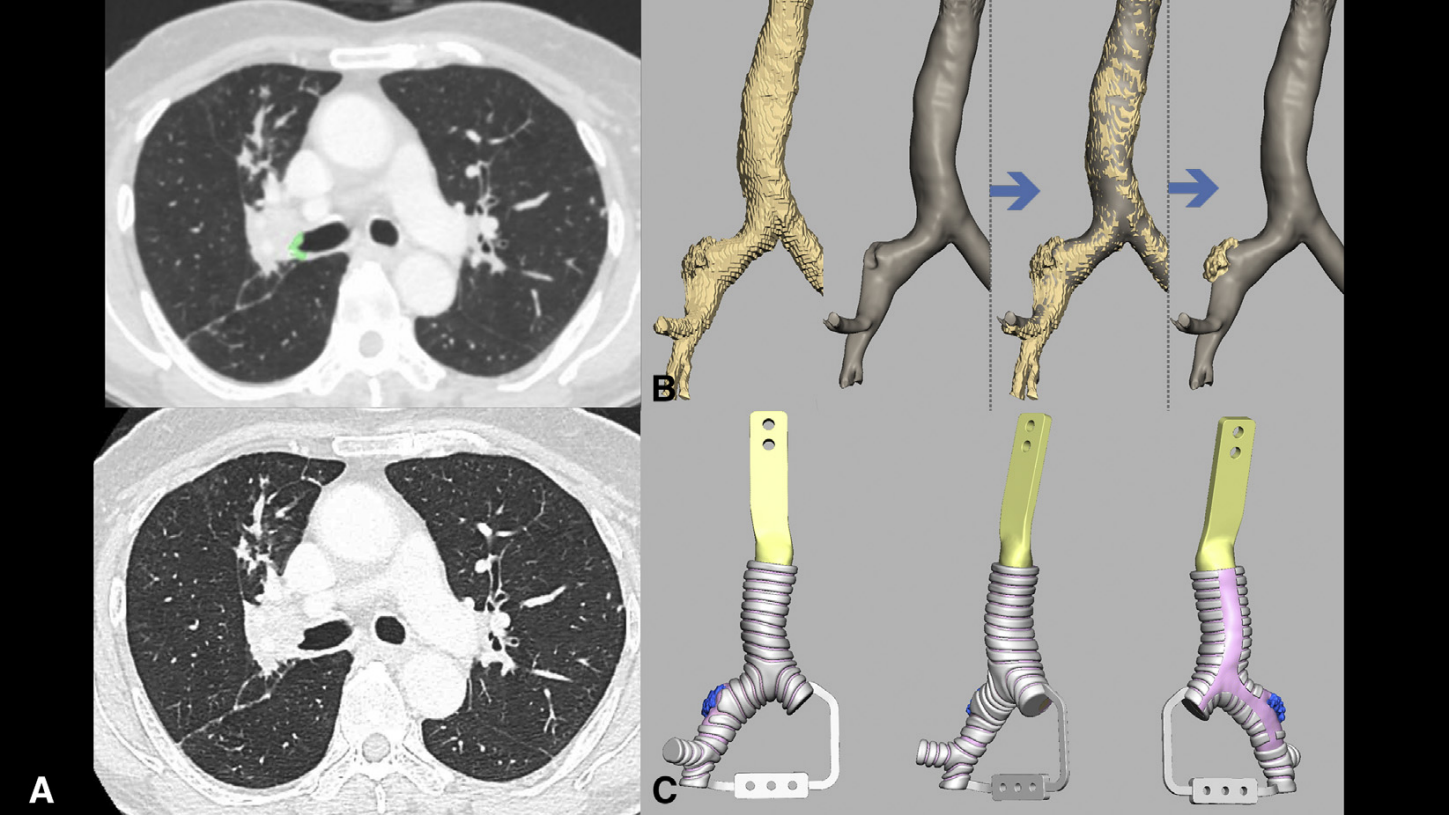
The sequence of the creation of the model (case 1: *right upper* sleeve lobectomy). A, *Upper*: The invasive area on the central airway was annotated (*green*) on preoperative chest computed tomography (CT) images. *Lower*: The same slice of the chest CT without annotation (raw CT data). B, The demarcation of the invasive lesion in the central airway by subtracting raw CT data from annotated CT data. C, The design of a 3-dimensional airway model using data obtained from chest CT. Note that the *pink-area* (connective tissue) is separately recognized from the *white area* (cartilage).
